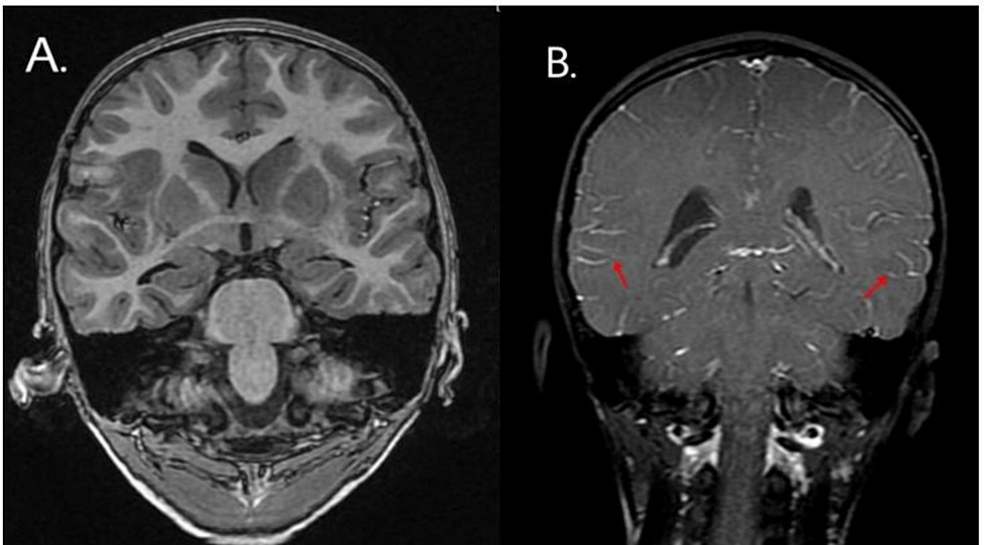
Coronal T1-weighted MRI of a six-year-old boy with COVID-19 encephalopathy (A) without contrast - normal and (B) with contrast - diffuse leptomeningeal involvement in bilateral cerebral hemispheres COVID-19: coronavirus disease 2019
